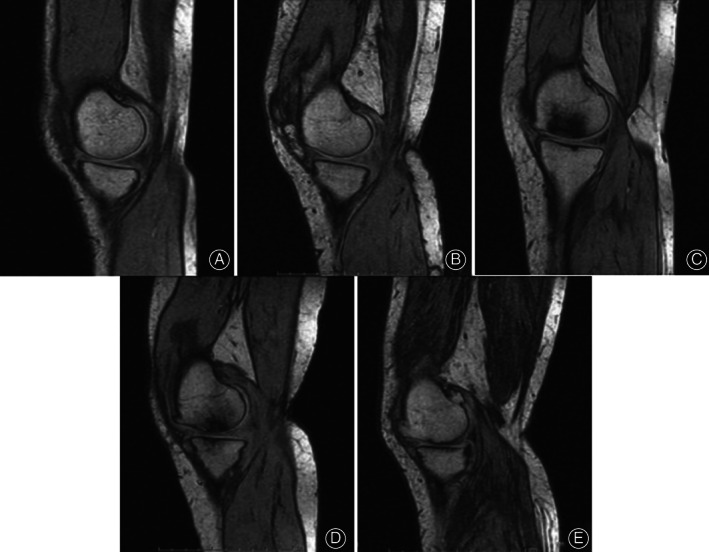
MRI T1WI for Grading of Knee‐joint Cartilage Injury. Grade 0: Normal, MRI indicates the joint cartilage signal was even, with normal thickness and smooth surface (A). Grade I: Joint cartilage was thickened, the signal for partial cartilage was decreased, the joint cartilage surface was smooth (B). Grade II: Local joint cartilage was irregular and coarse, and cartilage thickness was not apparently reduced (C). Grade III: The joint cartilage was irregularly thinned (D). Grade IV: Local joint cartilage has a defect, and partial subchondral bone was exposed (E).
